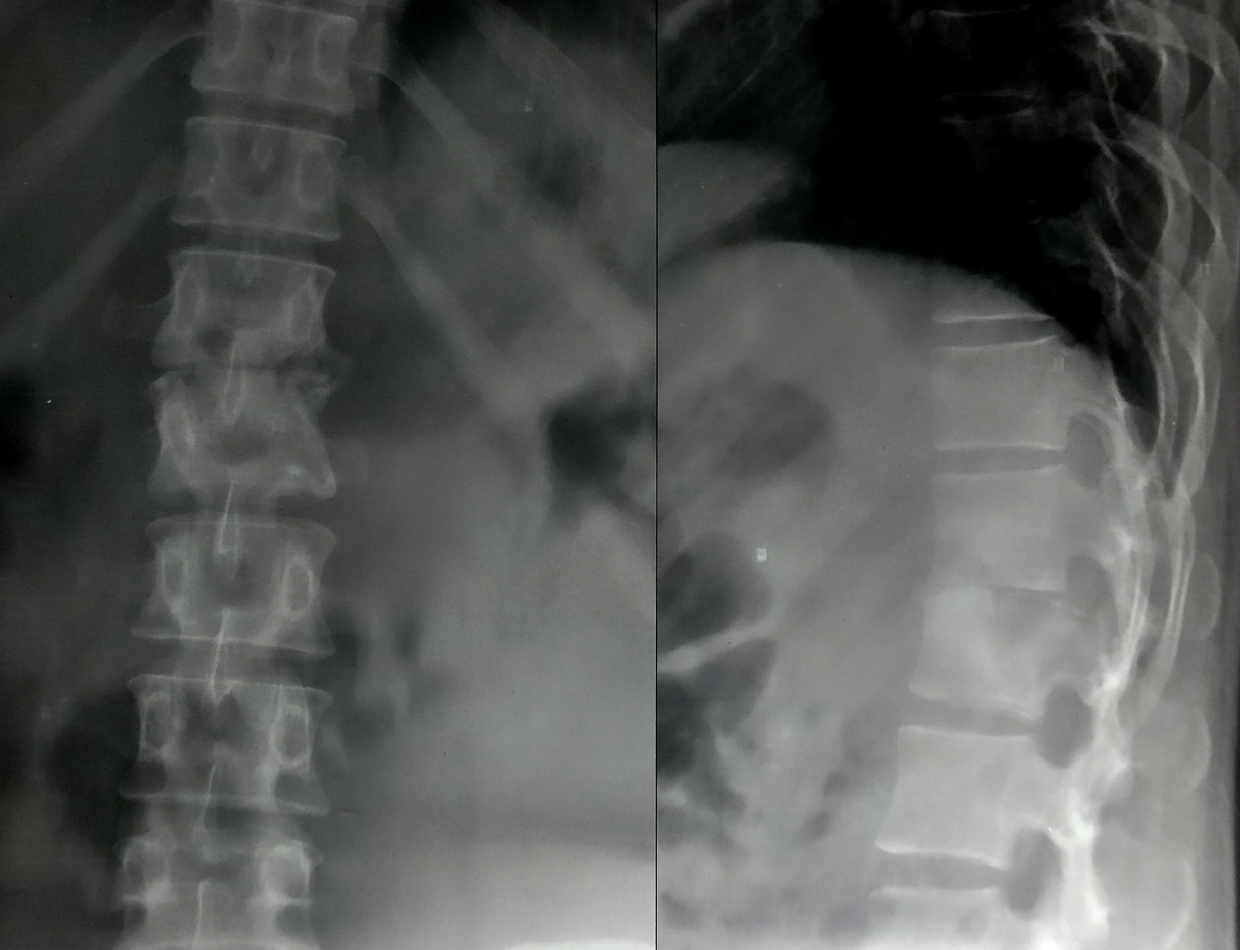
Pre-op radiograph of dorso-lumbar spine (antero-posterior and lateral views) Shows lesion at D12 and L1 vertebrae with kyphotic deformity and destruction of disc space.
